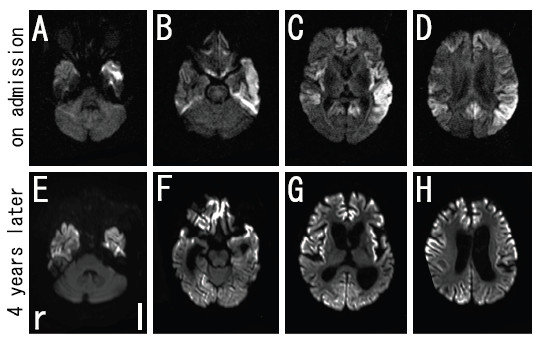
DWI images of brain MRI in the present case. Increased signal intensity in the bilateral frontal, temporal, and parietal cerebral cortex with left dominancy, except for occipital lobe, was observed on admission with axial imaging (A-D). Note that the high-signal region spread to the occipital cerebral cortex at four years after the onset (E-H).
